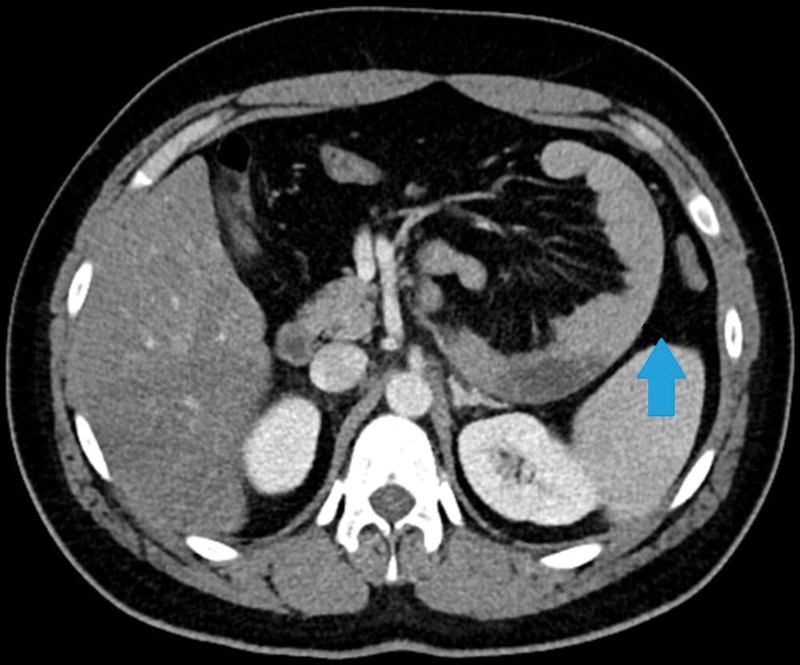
Axial CECT abdomen section showing abnormally located jejunum in left anterior pararenal space (blue arrow). CECT, contrast-enhanced computed tomography.
